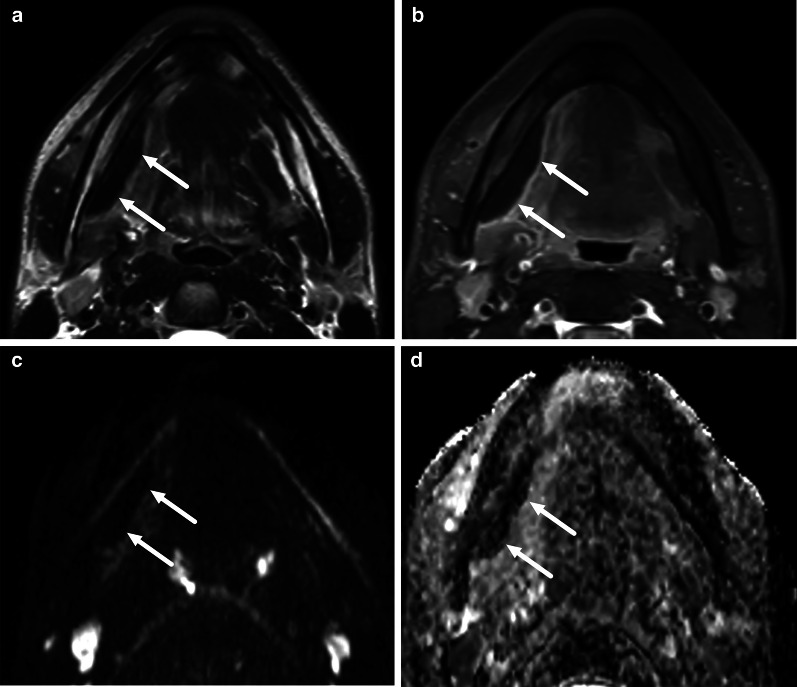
Subperiosteal hematoma after oral surgery (arrows). The collection is very hypointense on the axial T2-weighted image (a) which would be unusual for an abscess but mimics an abscess on the post-contrast fat-suppressed T1-weighted image (b). DWI of a hematoma can be misleading: the DWI trace image (c) shows T2 dark-through effects, whereas the ADC of the collection (d) looks artifactual and not like a true abscess. Surgery found hematoma
